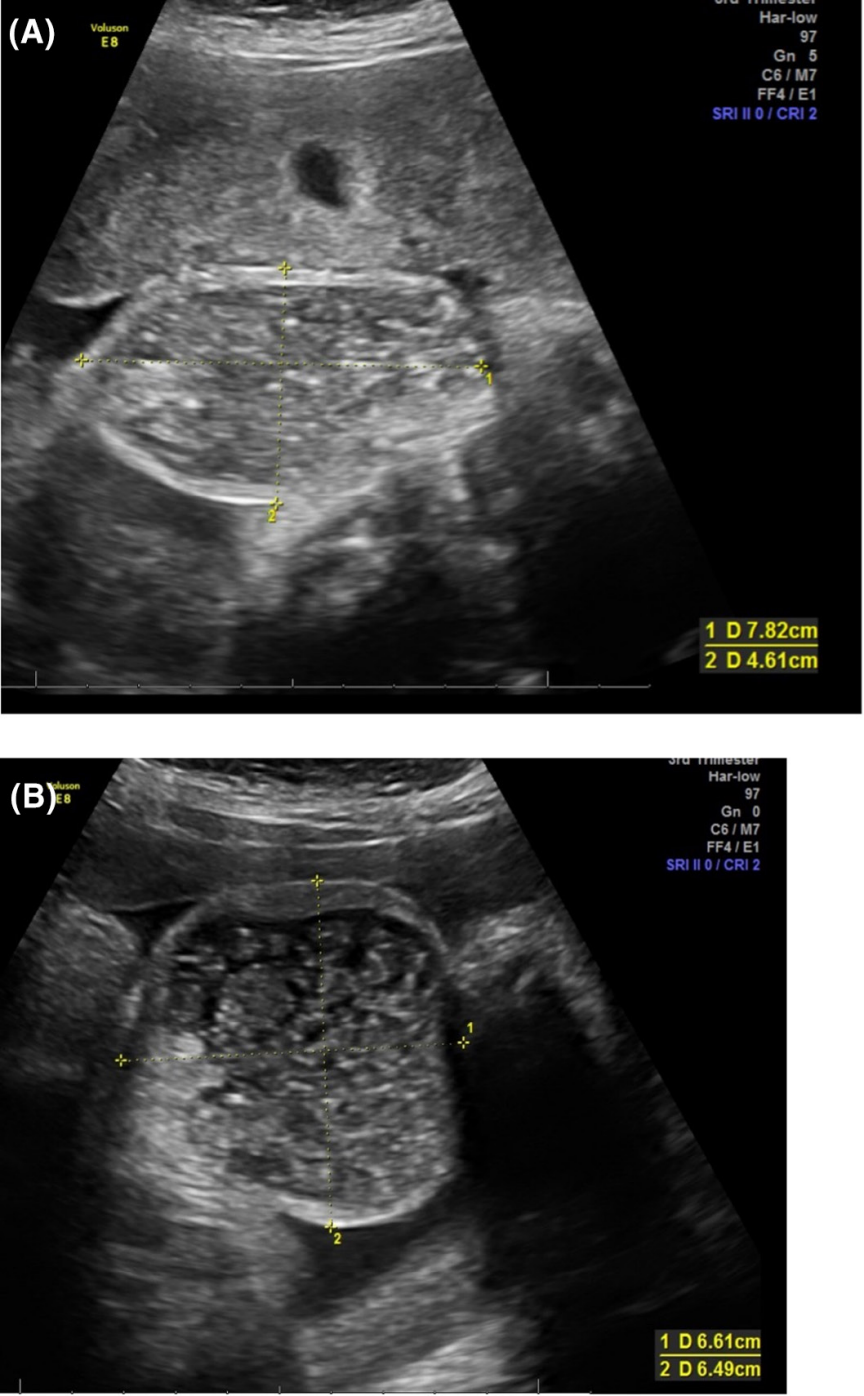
(A, B) Ultrasound images of an enlarged fetal scrotum (A: coronal plane, B: axial plane) filled with echogenic fluid, debris, and calcifications.
